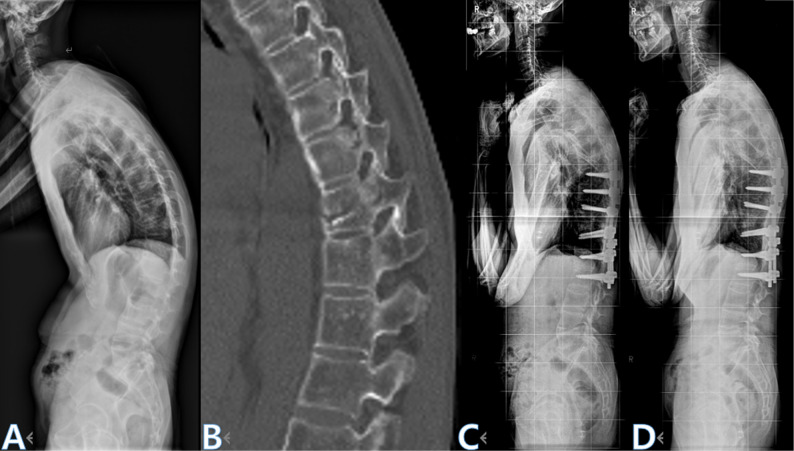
Representative case of a vertebral body (VB-type) fracture treated with PSO. A Preoperative lateral radiograph demonstrating sagittal malalignment. B CT scan showing a 3-column vertebral body fracture at T11. C 3-month postoperative radiograph after PSO and fusion from T8–L2. D Final follow-up radiograph showing maintained correction and fusion
